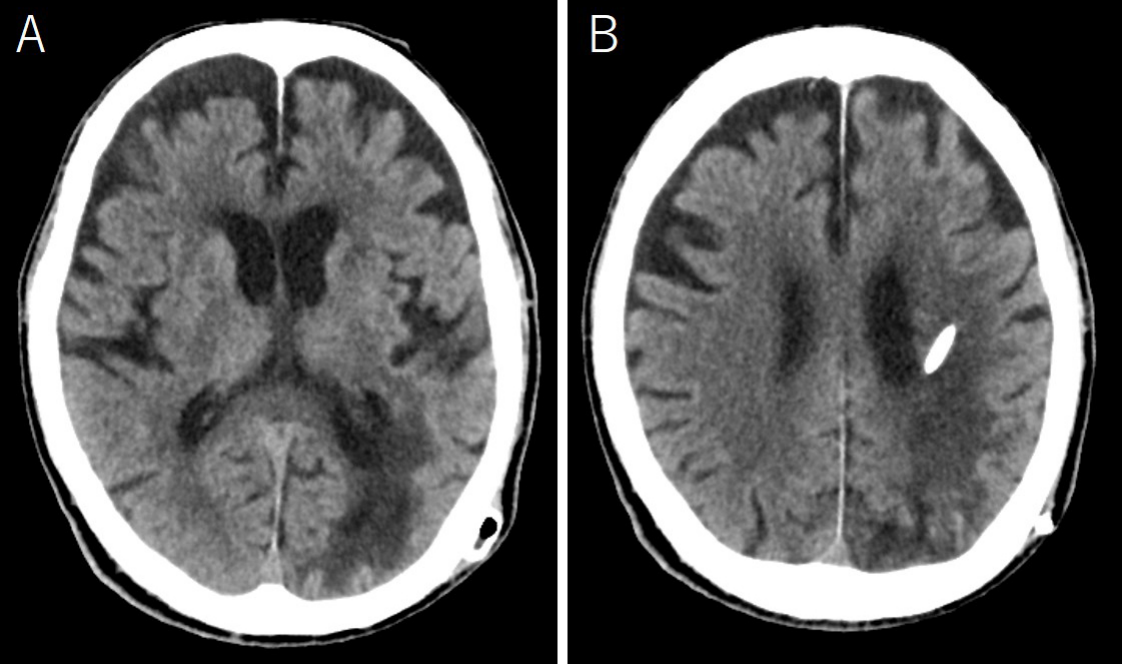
Computed tomography after the second operation revealed cyst shrinkage.
